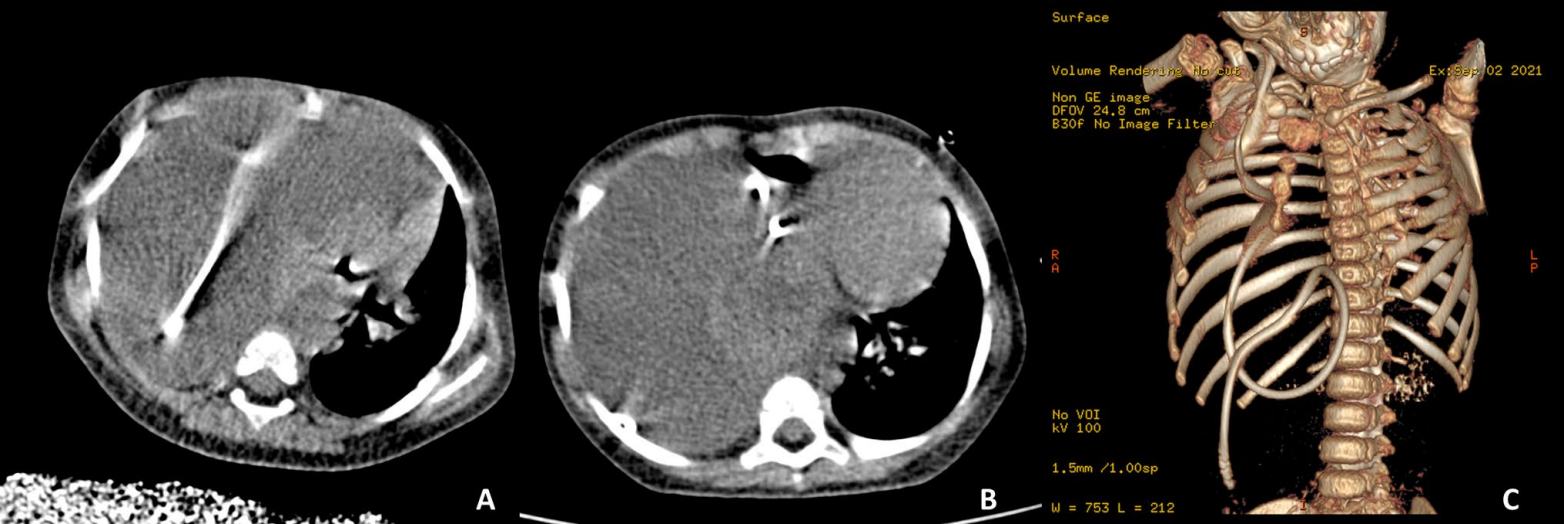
Preoperative chest CT scan demonstrating the massive pleural effusion, with the ventriculoperitoneal catheter visible inside the pleural cavity. A Before initial thoracostomy and (B) 2 weeks after thoracostomy. C 3d reconstruction at 2 weeks after thoracostomy
